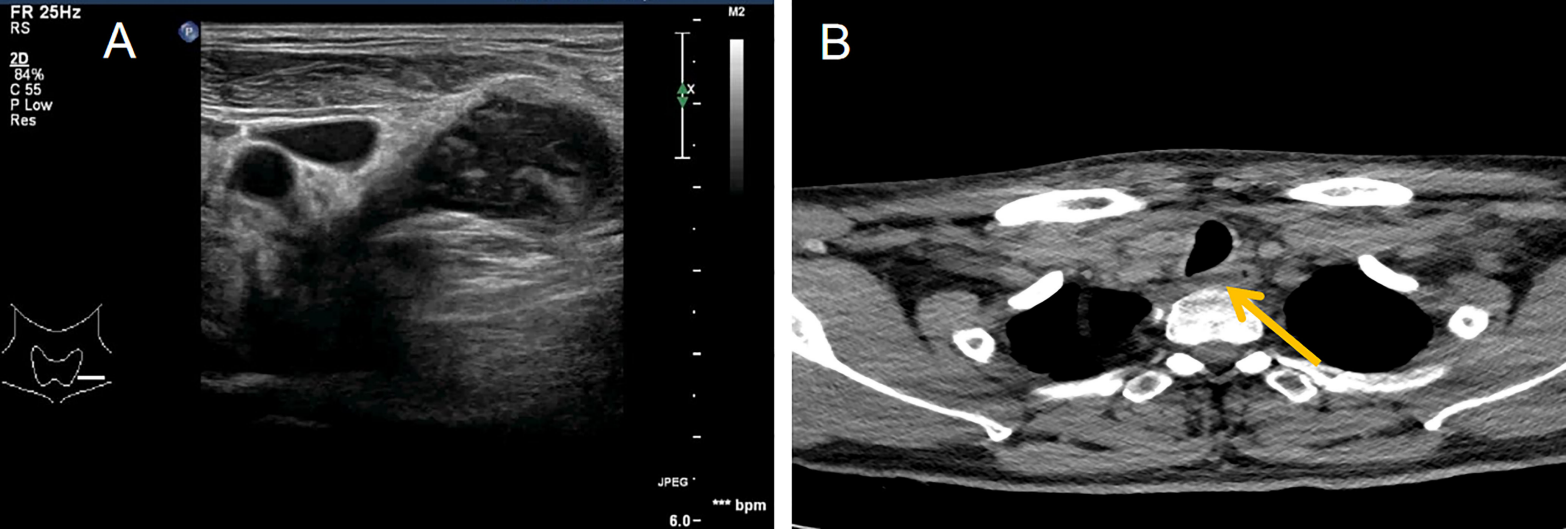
Ultrasound and computed tomography showing the posterior neck. (A) Ultrasound showing mixed echoes on the left side of the neck, poorly demarcated from the surrounding soft tissue and appearing to extend deep into the neck. This presentation is similar to the formation of tuberculous sinus passages in lymph nodes. (B) Neck computed tomography showing streaky fluid density shadows in front of the cone (yellow arrow points).
